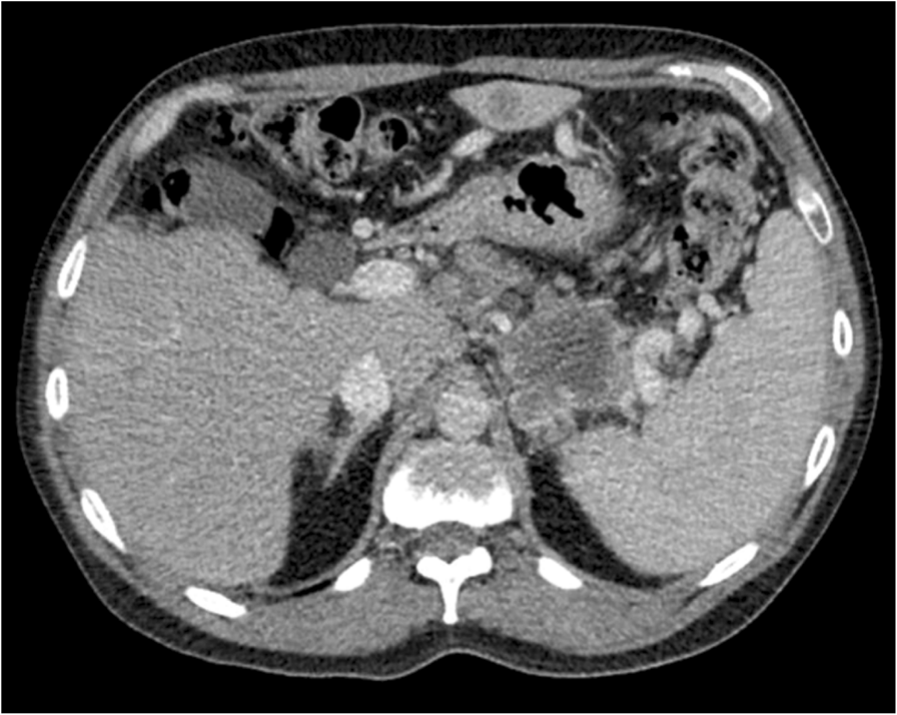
Axial plane abdominal/pelvic computed tomographic scan with intravenous contrast showing a 6.4 × 4.9-cm pancreatic tail mass inseparable from the left adrenal gland
